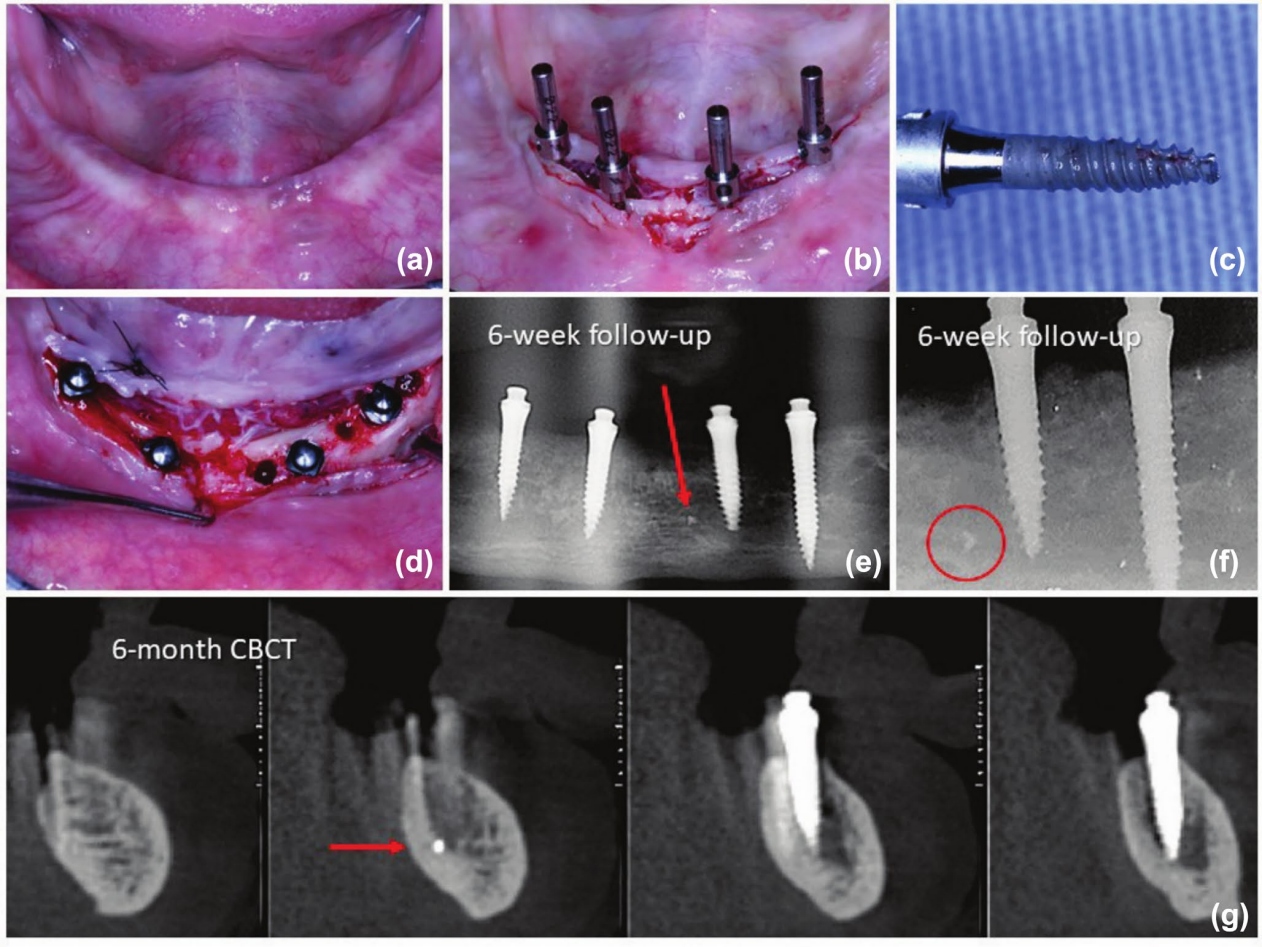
(a) Pre surgery view; (b) Initial transoperative view using a flapped approach; (c) Mini implant tip fracture; (d) Trans operative view showing the initial drilled sites and the final positions of the mini‐implants; (e) Panoramic radiograph at 6‐week follow‐up showing the fractured fragment; (f) Periapical radiograph of right implants at 6‐week follow‐up evidencing the fractured fragment; (g) CBCT at 6‐month follow‐up confirming the osseointegration of the implant and the fractured fragment.
